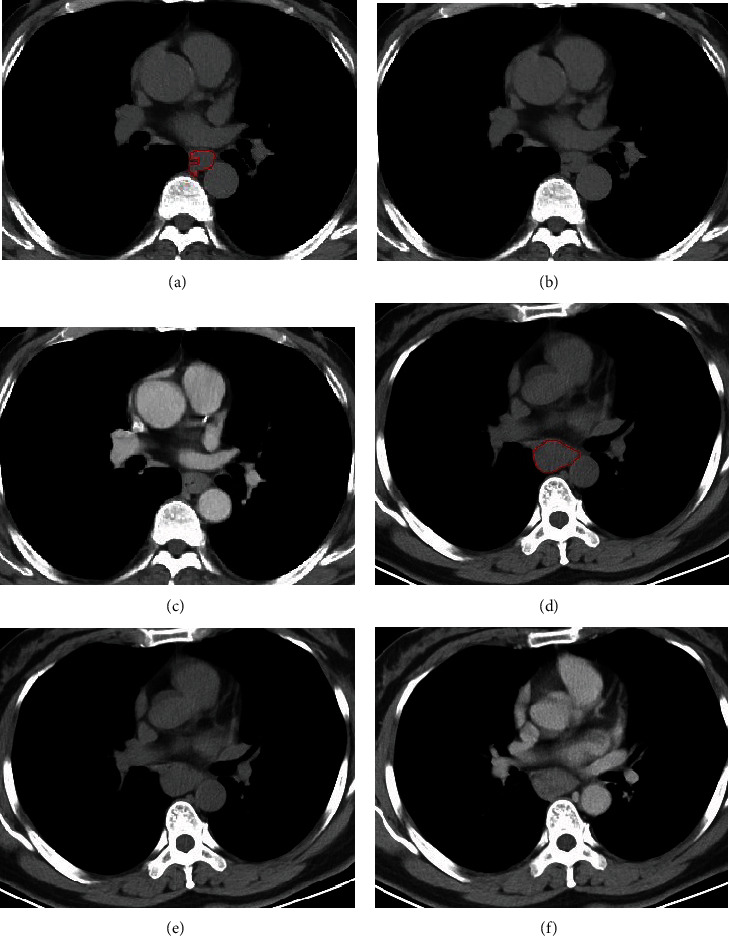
The cases of ROI segmentation and CT-based radiomics models for diagnosing ESCC and PMME. (a–c) 60-year-old man with ESCC. (a) The ROI was drawn along the tumor boundary in plain CT; (b) plain CT and (c) enhanced CT. Radiomics signature with score of 0.22 (less than the cutoff value of 0.50) suggested the diagnosis of ESCC. The radiomics nomogram model showed the radiomics score of 0.22 corresponded to 28 points, and the probability of diagnosing PMME was less than 0.01 indicating the diagnosis of ESCC. (d–f) 46-year-old women with PMME. (d) The ROI was drawn along the tumor boundary in plain CT; (e) plain CT and (f) enhanced CT. Radiomics signature with score of 0.98 (larger than the cutoff value of 0.50) suggested the diagnosis of PMME. The radiomics nomogram model showed the radiomics score of 0.98 corresponded to 98 points, and the probability of diagnosing PMME was larger than 0.99 stating the diagnosis of PMME.
